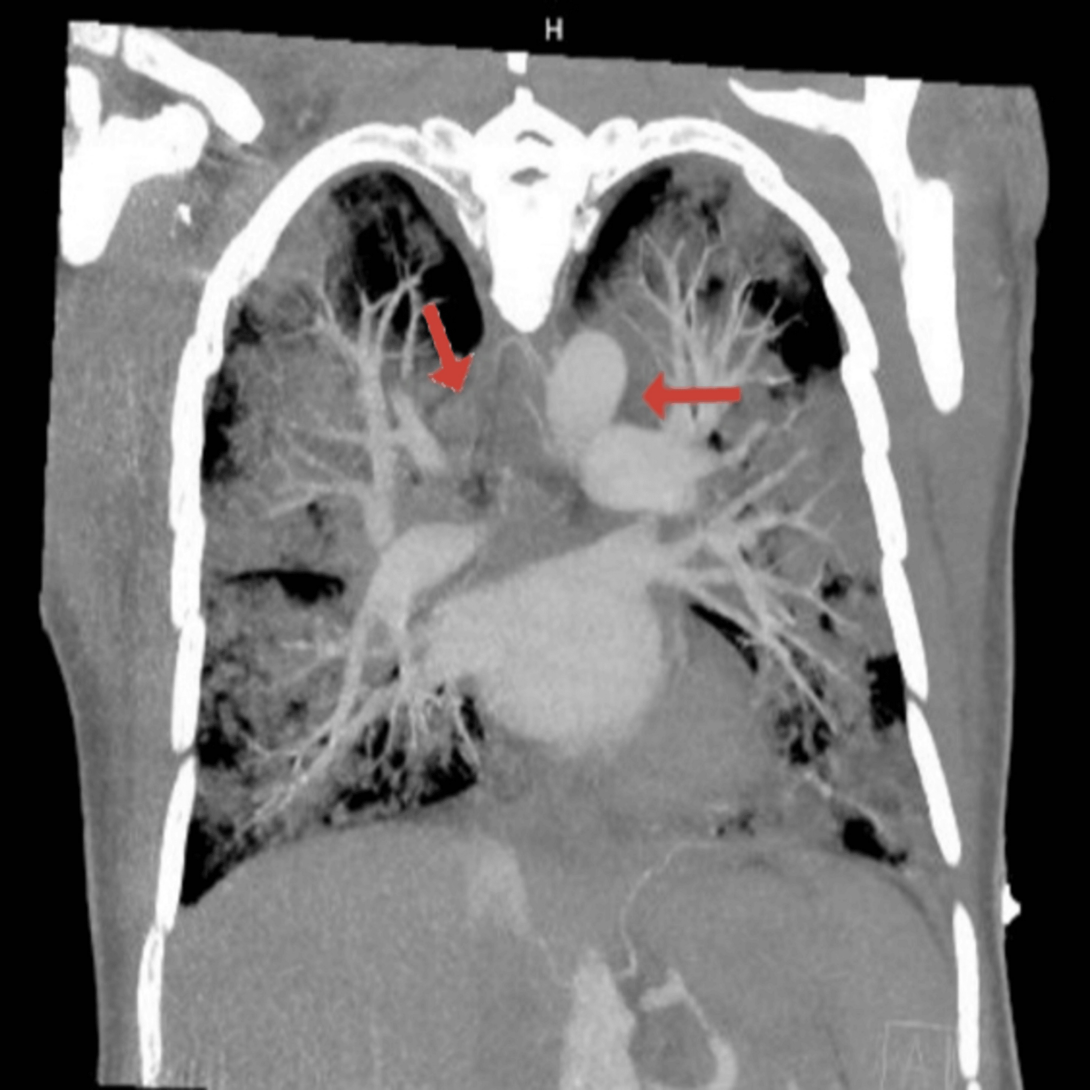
CT chest with angiography The image shows bulky mediastinal, prevascular, and axillary adenopathy; diffuse moderate to severe multifocal pneumonia throughout the bilateral lungs; and a small right-sided pleural effusion (arrows) CT: computed tomography
